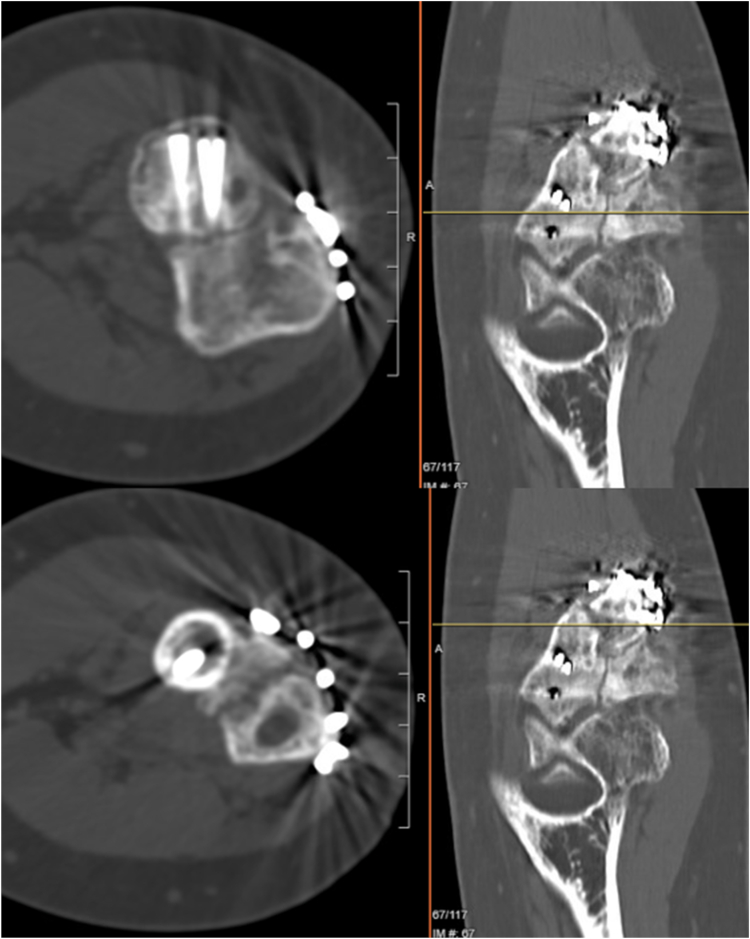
One-bone forearm revision postoperative computed tomography scan demonstrating callus formation and fusion at the revision site at 10 weeks.
